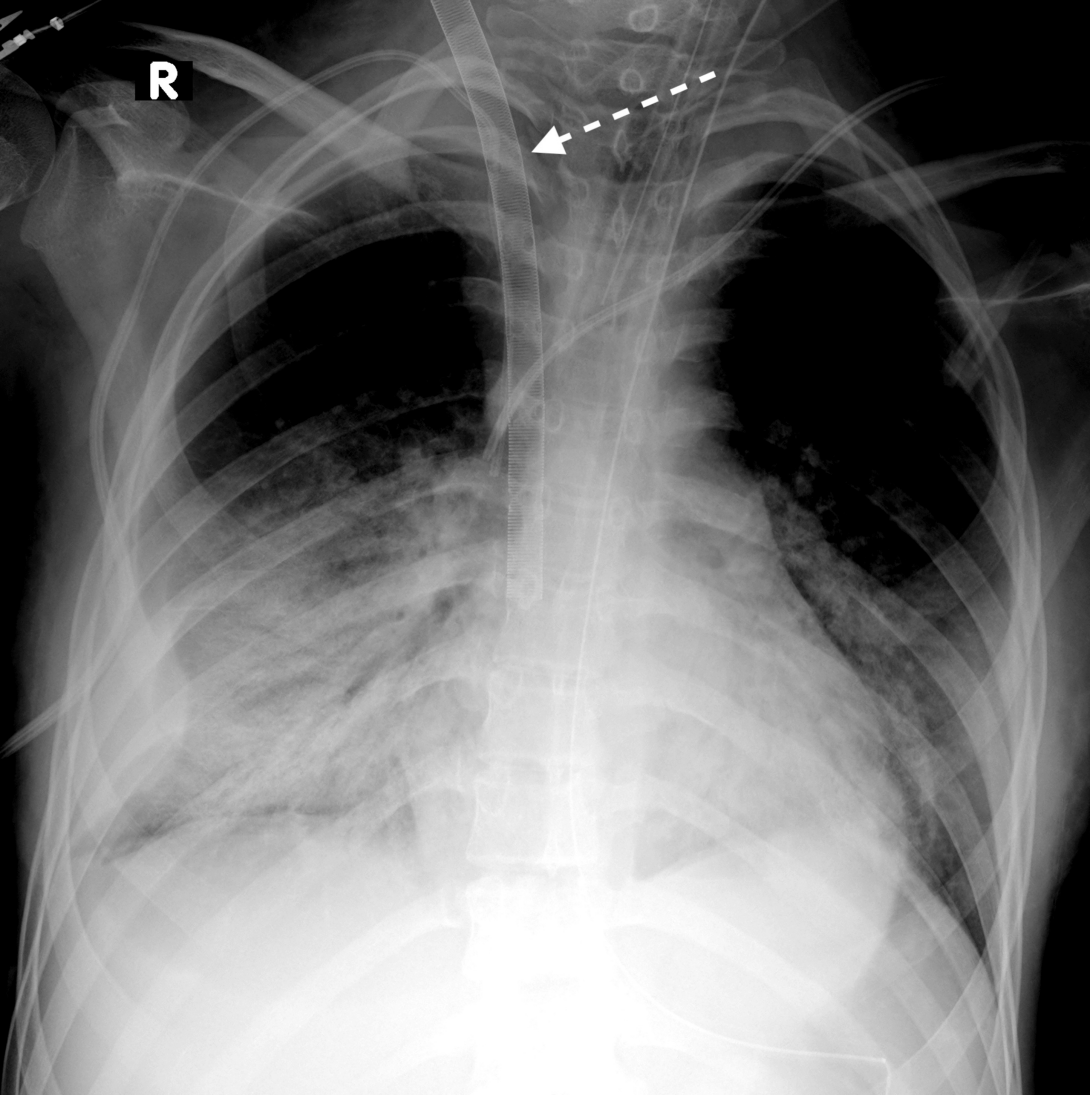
The X-ray after cannula retraction The first side holes are positioned above the clavicle.
